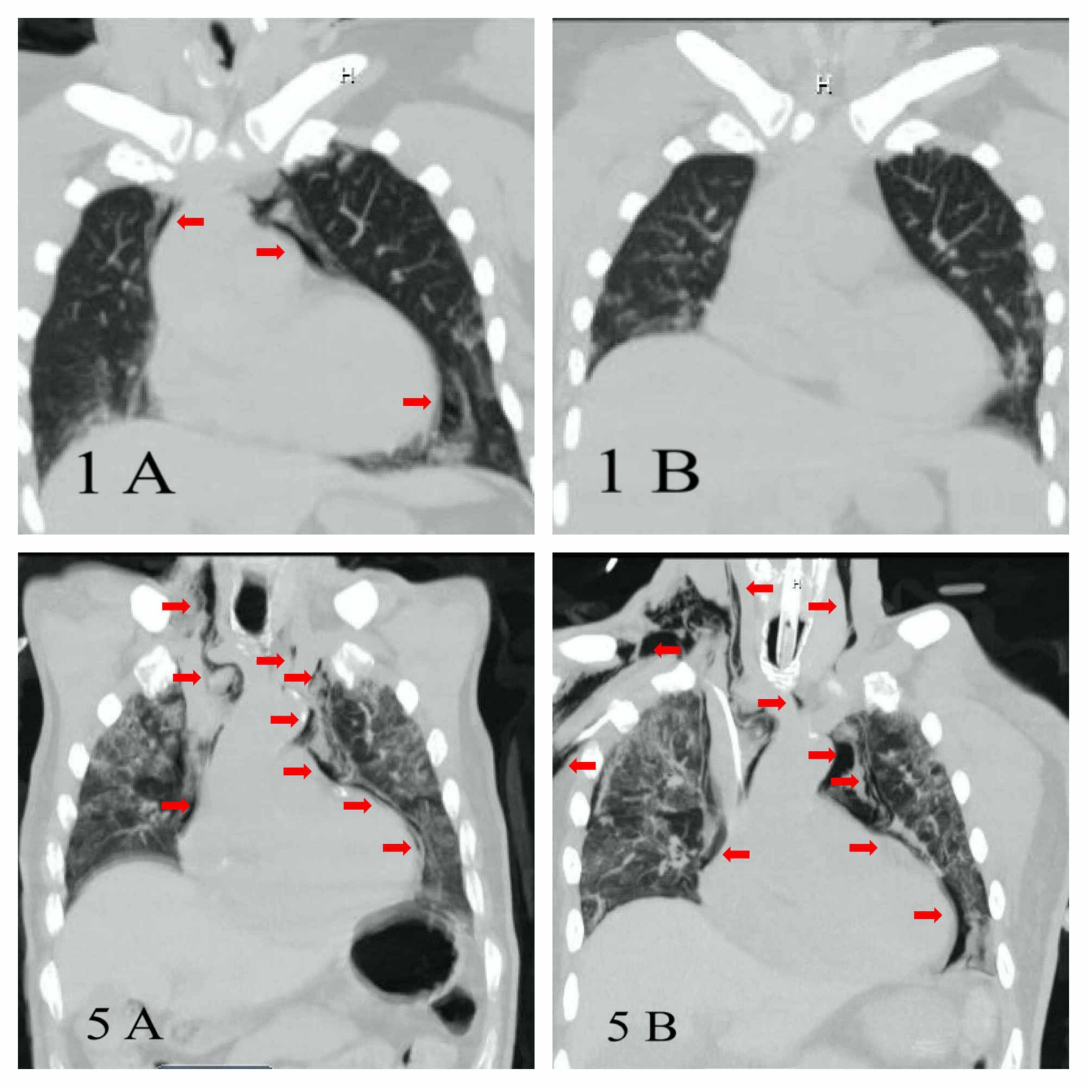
Computed tomography (CT) of the chest for patient 1 and patient 5 showing initial and follow-up imaging 1A - CT chest with pneumomediastinum extending into the subcutaneous emphysema in the neck and bilateral ground glass opacifications, 1B - CT chest showing resolution of the air leak, 5A - CT chest with pneumomediastinum tracking into the neck, 5B - CT chest post-intubation with severe worsening of the air leak
